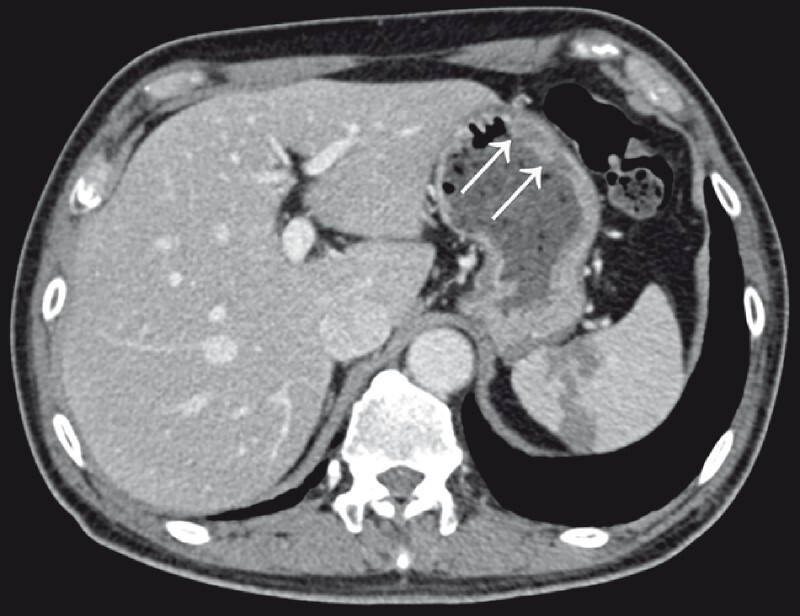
Enhanced computed tomography confirmed the presence of a low-density lesion in the stomach (arrows).
